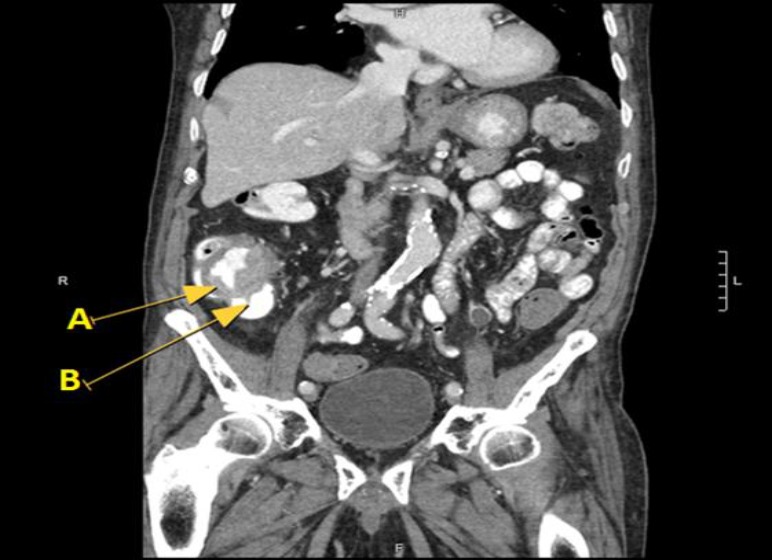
Coronal view, CT scan abdomen with contrast: Arrow ‘A’ pointing at malignant mass, which appears to encircle colonic wall to give an apple-core like lesion picture. Arrow ‘B’ is pointing at cecum
